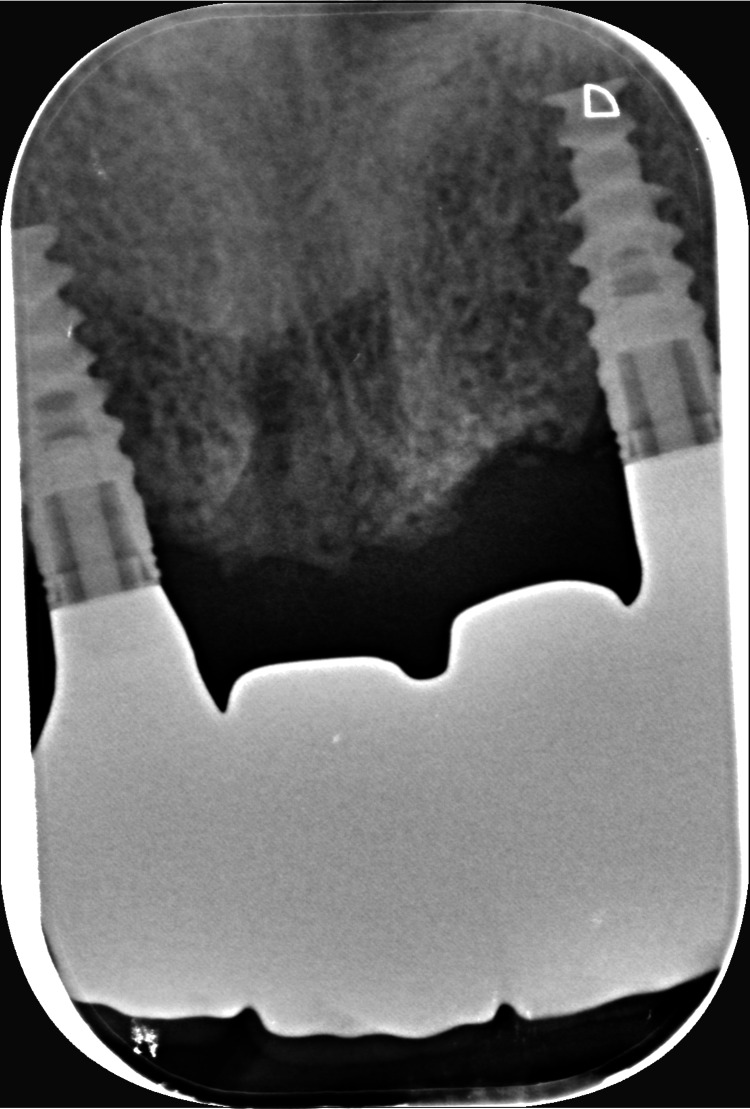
Intraoral radiograph of the framework screwed into implants #12 and #22
